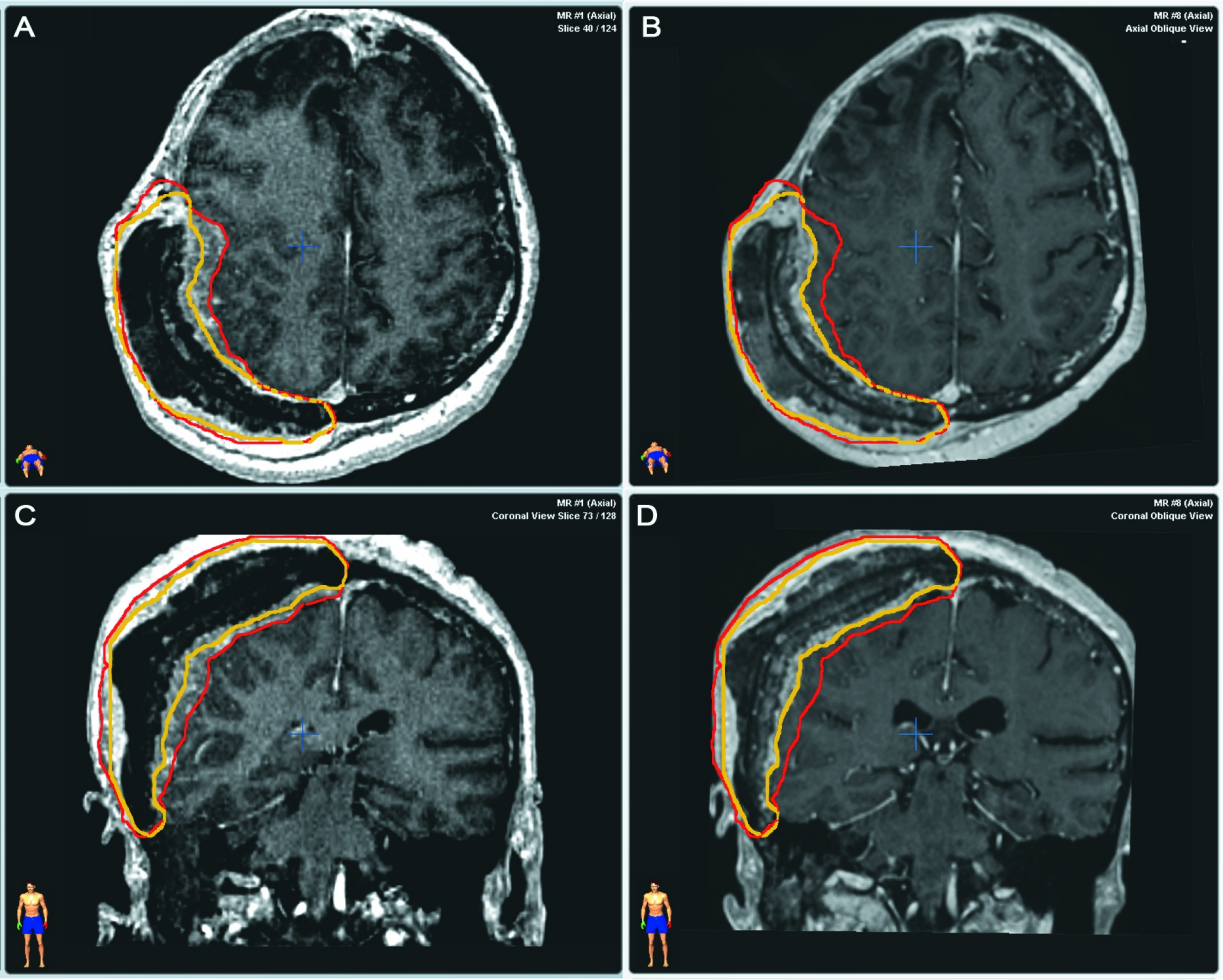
Tumor extent with MRI before and 6 years after irradiation Before irradiation - A (axial plane), C (coronal plane)/ 6 years after treatment - B (axial plane), D (coronal plane). Color lines delineate tumor contours before (red line) and after treatment (yellow line).
